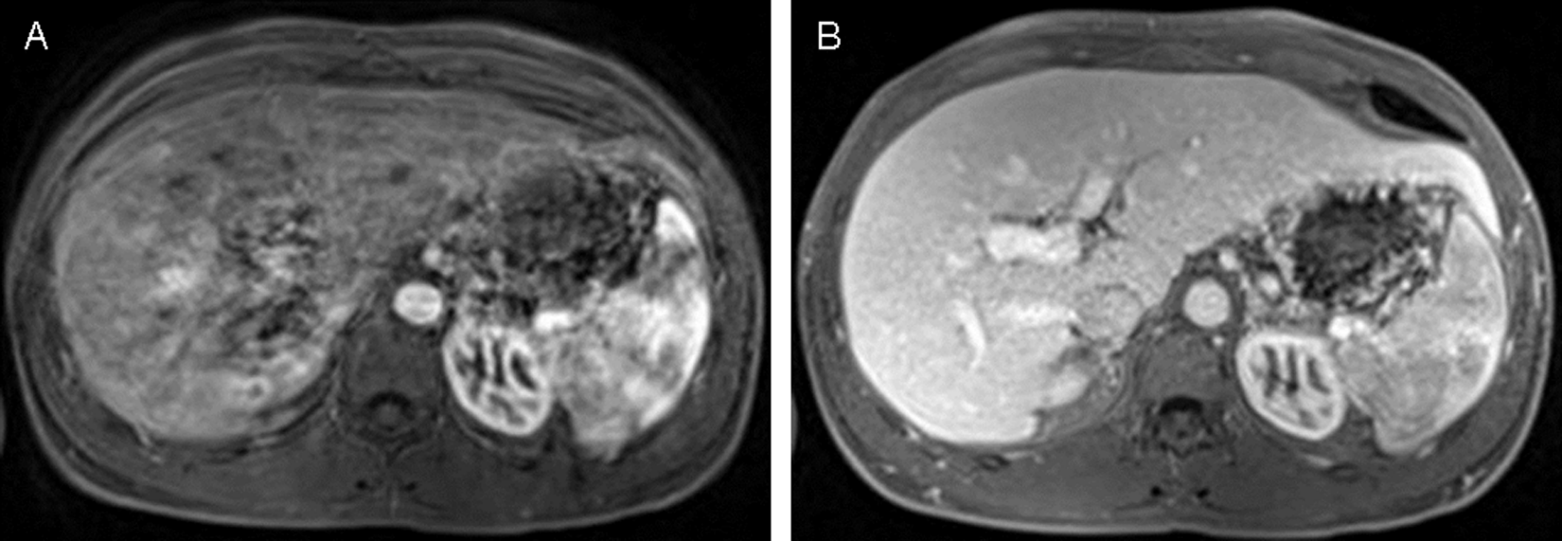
27 year old male patient with diagnosis of primary sclerosing cholangitis. Gadoxetate disodium-enhanced liver MRI in the arterial (A) and portalvenous phase (B). Self-limiting severe transient motion can be appreciated in the arterial phase, causing significant degradation of image quality, which is resolved in the portalvenous phase.
